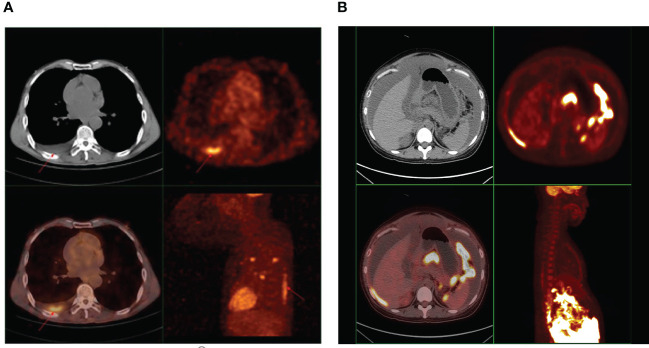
(A) A representative PET image showing ^18^F-FDG uptake in pleural effusion of cancer patients. A 58-year-old male diagnosed with adenocarcinoma of the lung, underwent whole-body PET/CT examination. The image showed ^18^F-FDG hypermetabolism in pleural effusion, and the SUV_max_ was 6.9. (B) A representative PET image showing ^18^F-FDG uptake in ascites of cancer patients. A 27-year-old female diagnosed with high-grade serous carcinoma of the ovary, underwent whole-body PET/CT examination. The image showed ^18^F-FDG hypermetabolism in ascites, and the SUV_max_ was 2.18.
